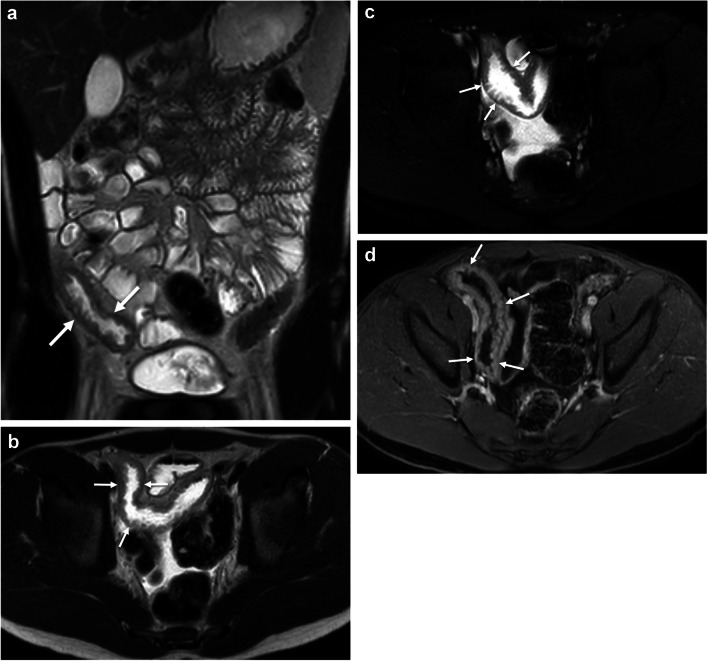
A 15-year-old with a family history of Crohn disease and new perianal drainage. A Coronal T2-weighted single-shot fast spin-echo, (B) axial T2-weighted single-shot fast spin-echo, (C) axial T2-weighted single-shot fast spin-echo fat-saturated, and (D) axial postcontrast T1-weighted MR images show findings consistent with ileal Crohn disease, including multiple radiologic ulcers (*arrows*) of variable depth and severe in extent (6 or more visible ulcers)
